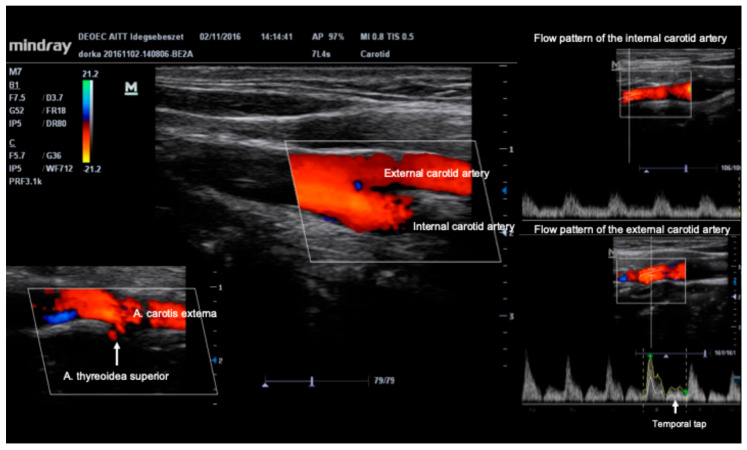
Differentiation between internal and external carotid artery using B-mode and colour-coded linear ultrasound.
